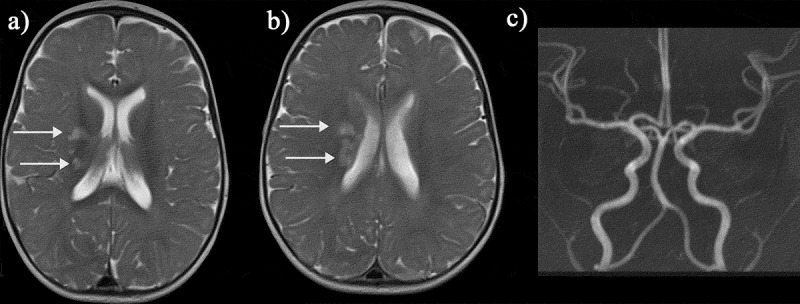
Basal ganglia infarct: This young child who is a compound heterozygote had a left hemiparesis at the age of six months, before transcranial Doppler screening was mandated. Prior to that, the child had had a cold on and off for the last few months and tended to breathe rather heavily and to snore on and off. (a,b) The T2-weighted magnetic resonance imaging showed patchy hyperintensity within the right superomedial striato-capsular region, with associated diffusion restriction (not shown), consistent with acute evolving infarction. (c) Magnetic resonance angiography was normal, as was imaging transcranial Doppler but patent foramen ovale was demonstrated on bubble contrast echocardiography although venography of the legs and pelvis did not demonstrate clot. Treatment was with aspirin as well as regular blood transfusion
